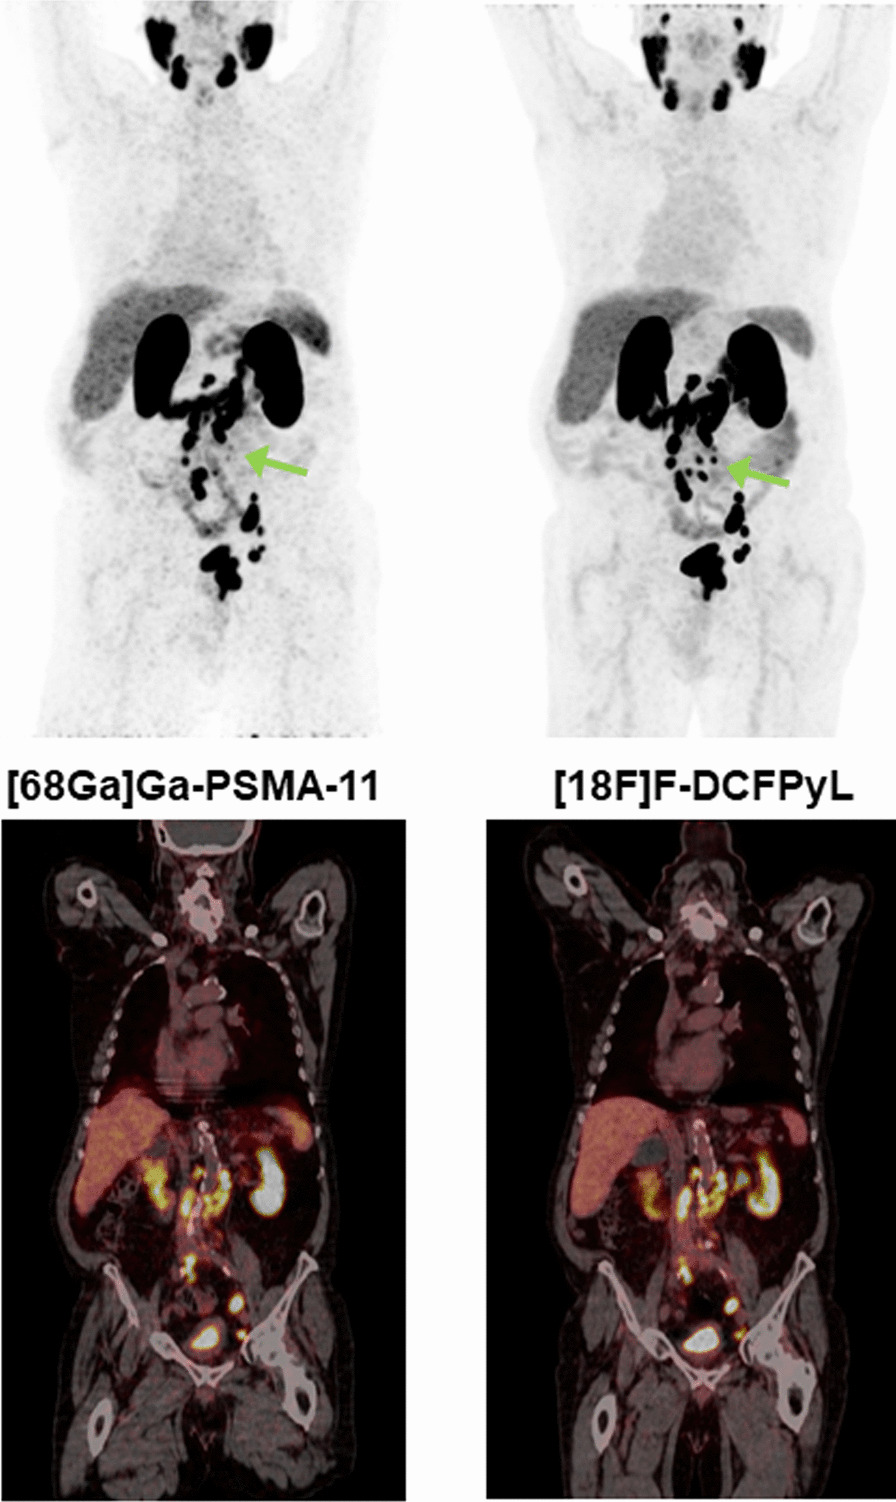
Image comparison of PET using [^68^Ga]Ga-PSMA-11 (left) and [^18^F]F-DCFPyL (right). PET images of patient no. 4 (lesions 6–12) are shown. Both PET with [^68^Ga]Ga-PSMA-11 and [^18^F]F-DCFPyL show evidence of local recurrence and extensive iliac and retroperitoneal lymph node metastases, as shown in the maximum intensity projection images (top row). The coronary fusion images (bottom row) show the bi-iliac and retroperitoneal lymph node metastases as expected, while the local recurrence is outside the imaged plane. Visual impression of tracer distribution confirms the statistically suspected comparability of tumour-to-liver ratios and tumour-to-mediastinum ratios between PET using [^68^Ga]Ga-PSMA-11 and [^18^F]F-DCFPyL. Furthermore, the well-known strengths of ^18^F-labelled PSMA tracers are apparent in the noticeable reduction in background noise and superior detectability of smaller lesions (green arrow) in the images from [^18^F]F-DCFPyL PET
